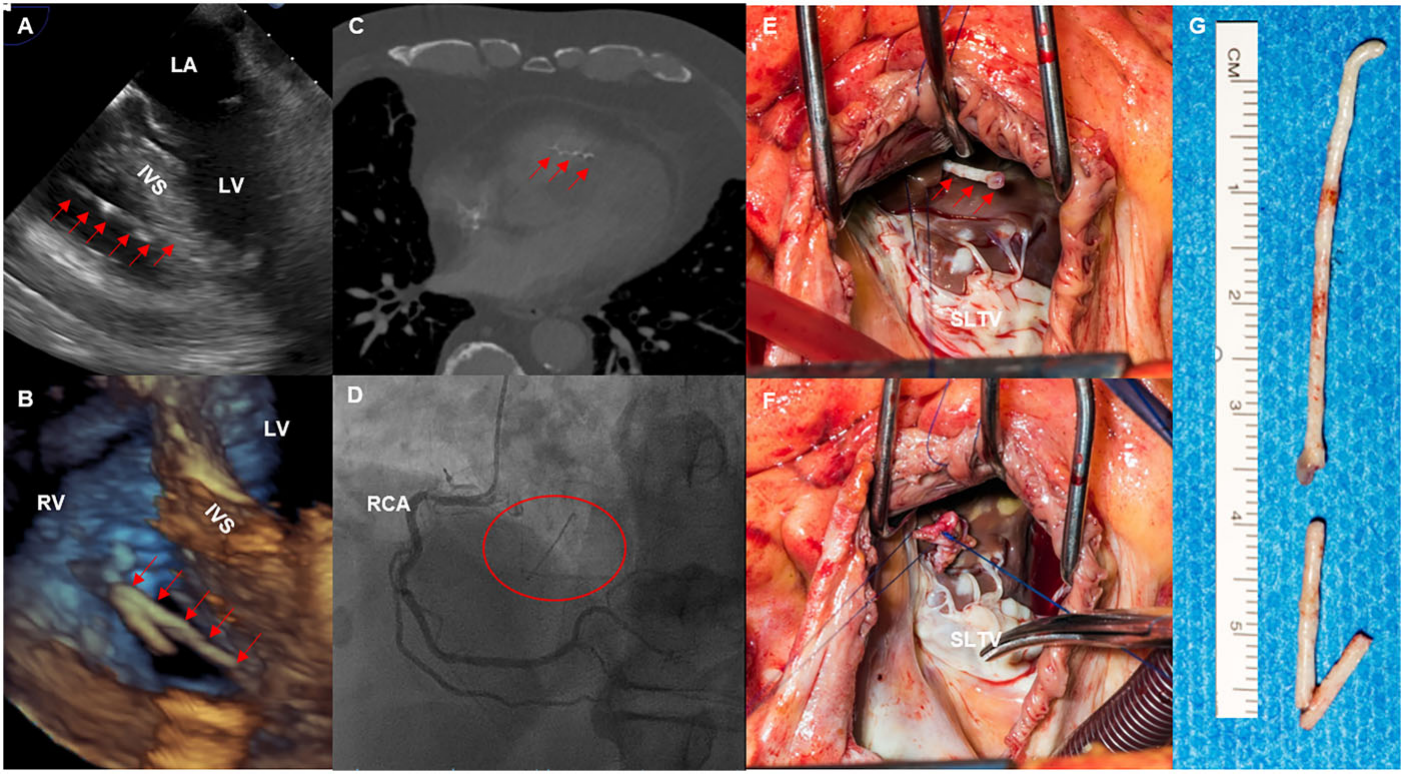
(A and B) Perioperative transoesophageal echocardiography showing the foreign body in the right ventricle, penetrating the interventricular septum (arrows). (C) Computed tomography scan showed a 6.5-cm-long cement fragment in the right ventricle. (D) Coronary angiogram, revealing the cement fragments in the right ventricle (circle). (E and F) Surgical view through the right atrium on the foreign body (arrows). After removal, the entry into the interventricular septum was closed, using a pericardial patch (septal leaflet of the tricuspid valve). (G) Bone cement after surgical removal.
